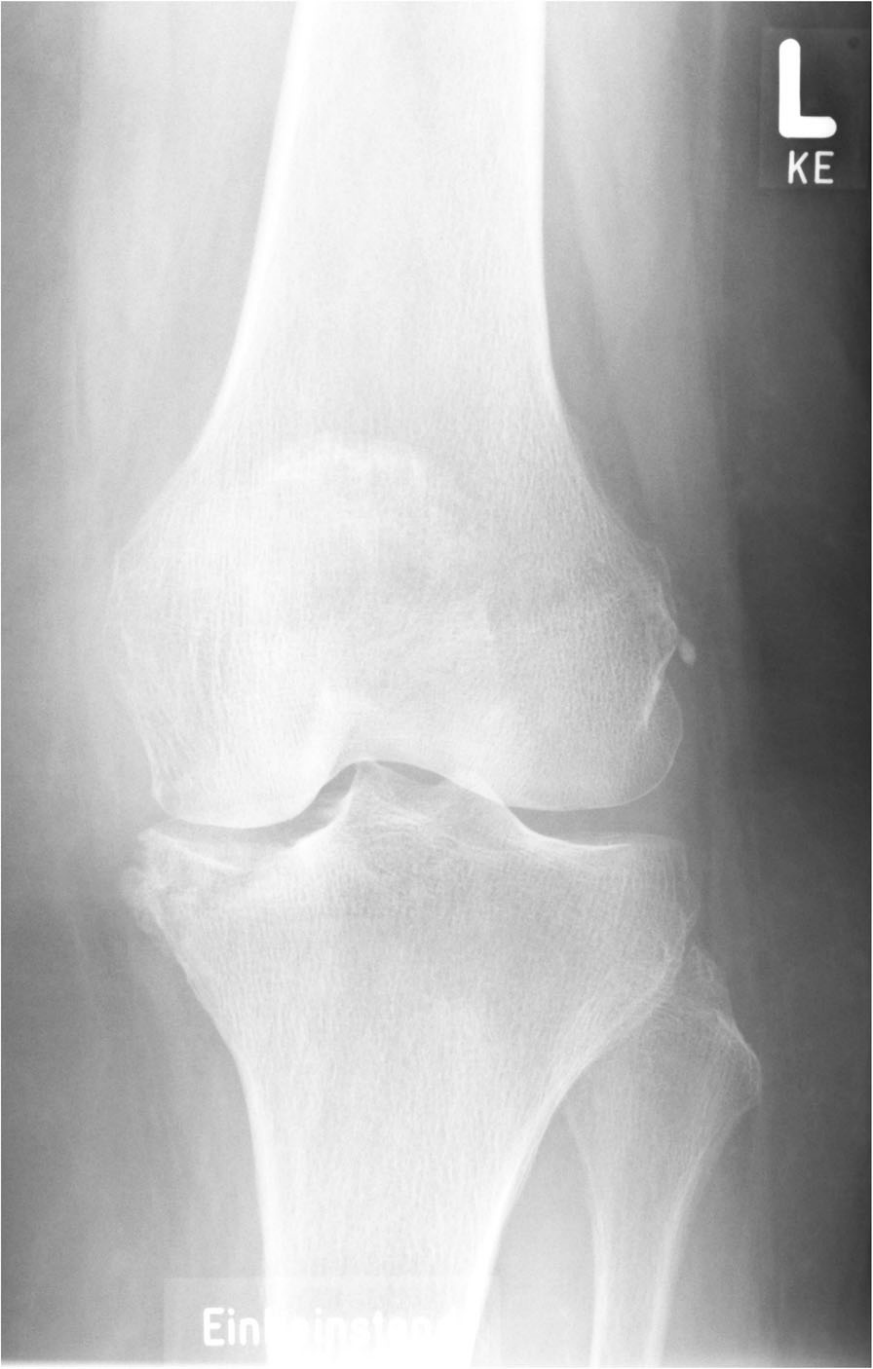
Case 1 patient: anterior-posterior radiography left knee: medial tibial plateau osteonecrosis, cystic demarcation. L = left, KE is no information concerning the patient
